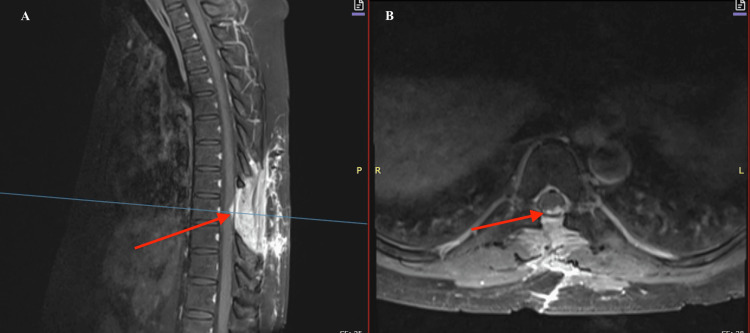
Three-month postoperative T1-weighted contrast-enhanced MRI of the thoracic spine Sagittal (A) and axial (B) T1-weighted contrast-enhanced MRI images showing postsurgical changes following T8 and T9 laminectomy and resection of the spinal cord tumor. Residual enhancement is present within the dorsal spinal cord at T9 (red arrows), measuring 0.5 × 0.8 × 1.3 cm.
